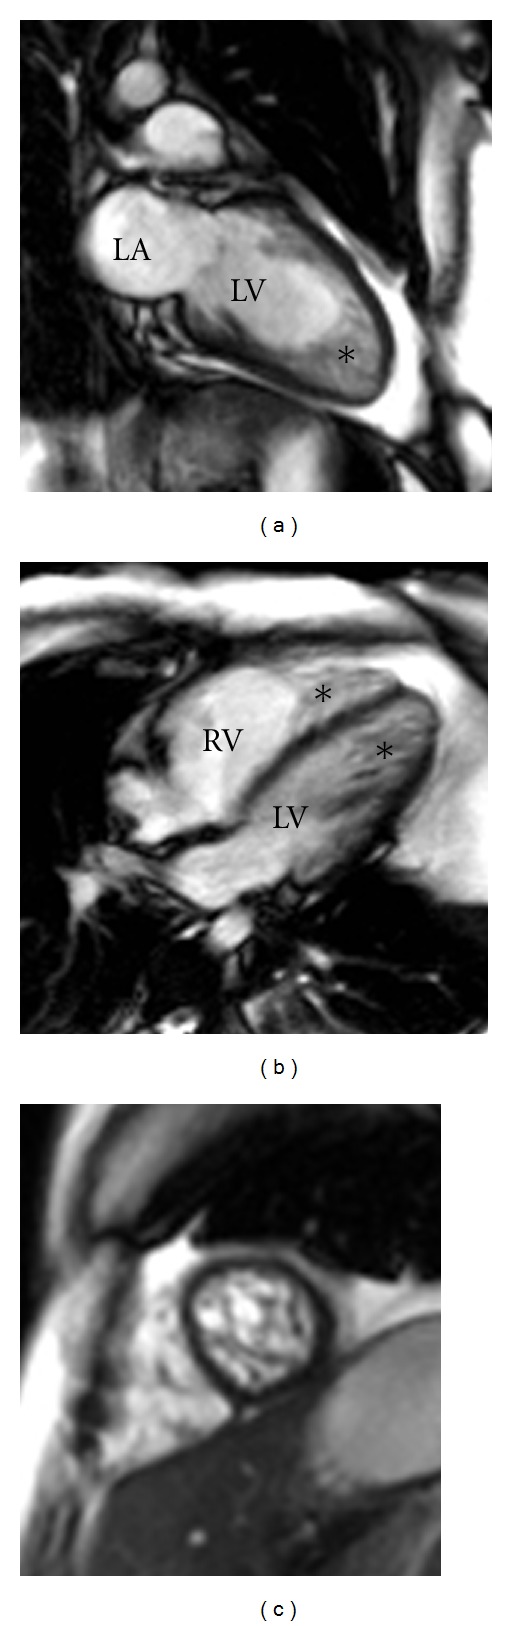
Cardiac MRI of the heart. ((a) and (b)) Cardiac MRI of the LV showing prominent muscular trabeculations (asterisk) and spongiform appearance of the ventricular cavities. (c) Short axis cardiac MRI image through the distal RV and LV, demonstrating trabeculations filling the ventricular cavity.
